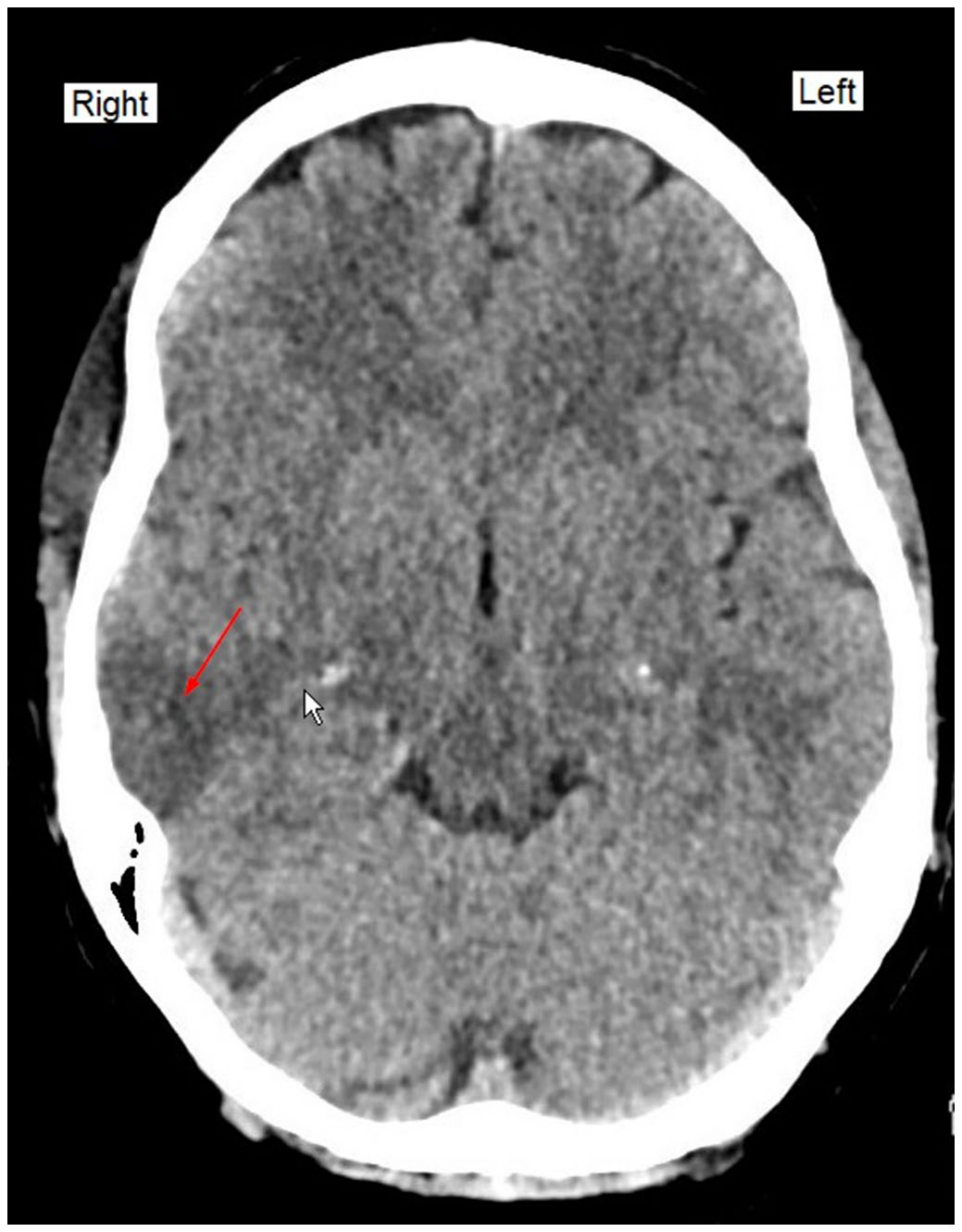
CT (Computed Tomography) of the brain on admission. Red arrow indicate the possible minor acute or subacute infarcts in the right temporal lobe.
